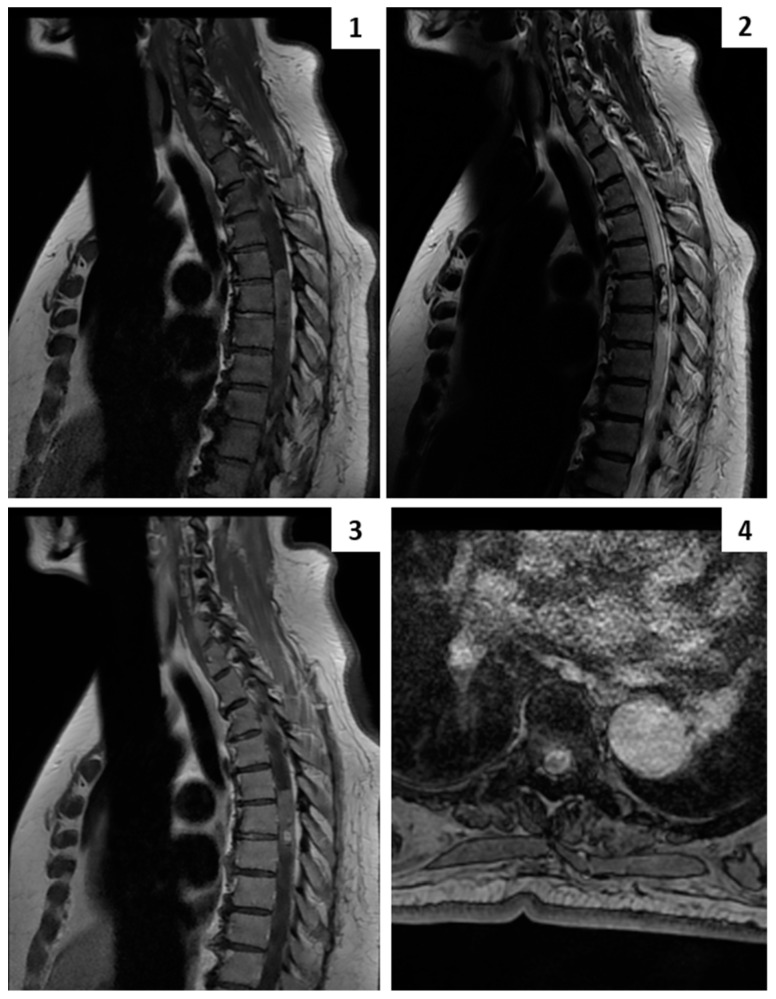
Thoracic preoperative MRI. (1) sagittal T1-weighted sequence, (2) sagittal T2-weighted sequence, (3) sagittal contrast-enhancing T1-weighted sequence, (4) axial T1-weighted BRAVO sequence show a thoracic intramedullary tumor extending from Th5 to Th7characterized by hyperintensity in T1-weighted images, an enhancing nodule in CE T1-weighted images, and a heterogeneous aspect in T2-weighted images.
